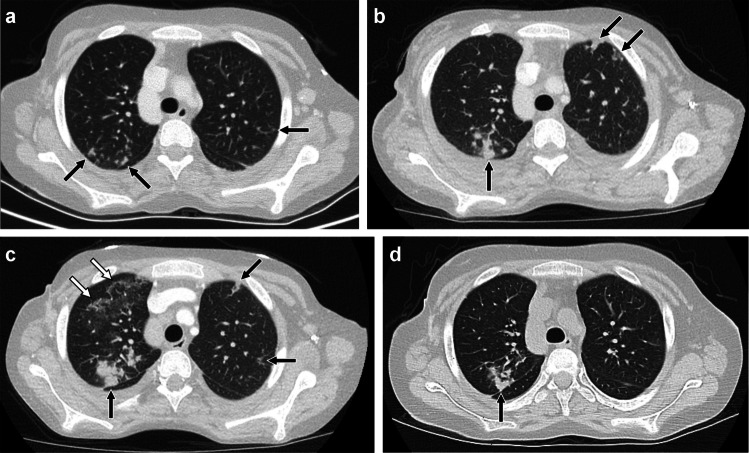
A 17-year-old female with epidermolysis bullosa who developed asymptomatic waxing and waning opacities on chest CT while receiving cemiplimab (an immune checkpoint inhibitor targeting programmed death receptor-1) for squamous cell carcinoma. Bronchoscopy with bronchoalveolar lavage and transbronchial biopsy demonstrated increased macrophages without evidence of infection, malignancy, or vasculitis. a-c Axial images from three chest CTs with contrast obtained over the course of 4 months showing waxing and waning/migratory upper lobe peripheral predominant nodular foci of consolidation (*black arrows*) as well as perilobular and ground-glass opacities (*white arrows*) suggesting an organizing pneumonia pattern. d Axial CT image without contrast obtained 1 month later, after a course of steroids for suspected immune checkpoint inhibitor-induced lung disease, showing improvement in bilateral upper lobe findings from the most recent prior chest CT, with decreased nodular consolidation remaining in the right upper lobe (*black arrow*)
